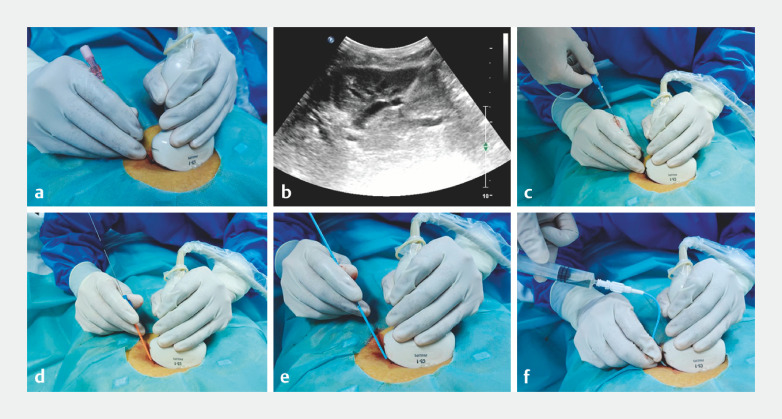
Ultrasound-guided percutaneous transhepatic puncture of the left extrahepatic bile duct.
